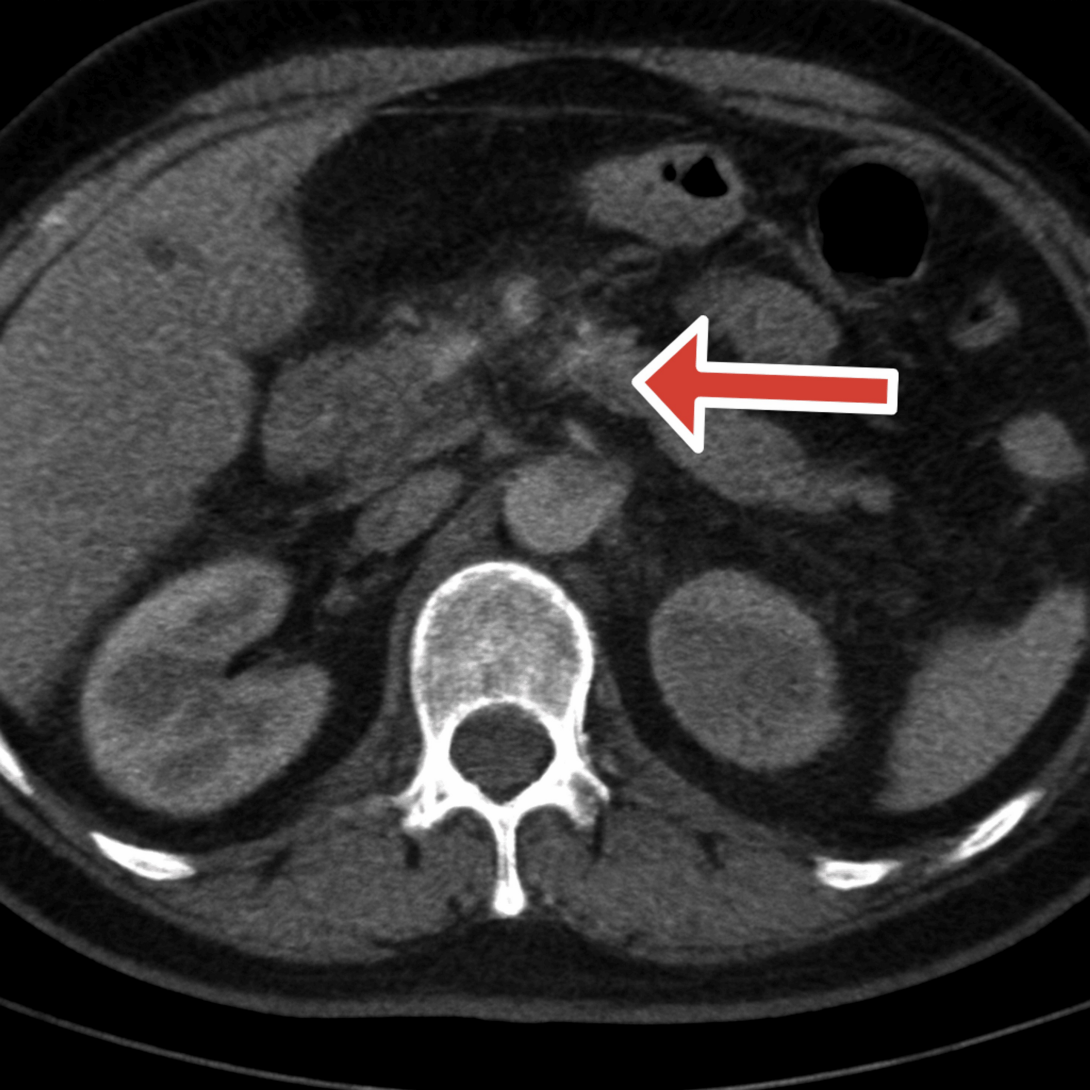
Contrast-enhanced CT of the abdomen and pelvis showing necrotic pancreatic parenchyma (Oct 24, 2024) CT: Computerized tomography The red arrow shows a necrotizing pancreas with mild fluid. Modified CTSI (Computed Tomography Severity Index): Pancreatic inflammation: Intrinsic pancreatic abnormalities with peripancreatic inflammation (Score: 2). Pancreatic necrosis: Approximately 30–50% non-enhancing parenchyma (Score: 4). Extrapancreatic complications: Presence of peripancreatic fluid collections (Score: 2). Total Modified CTSI Score: 8/10. Severity Grade: Severe Acute Necrotizing Pancreatitis
